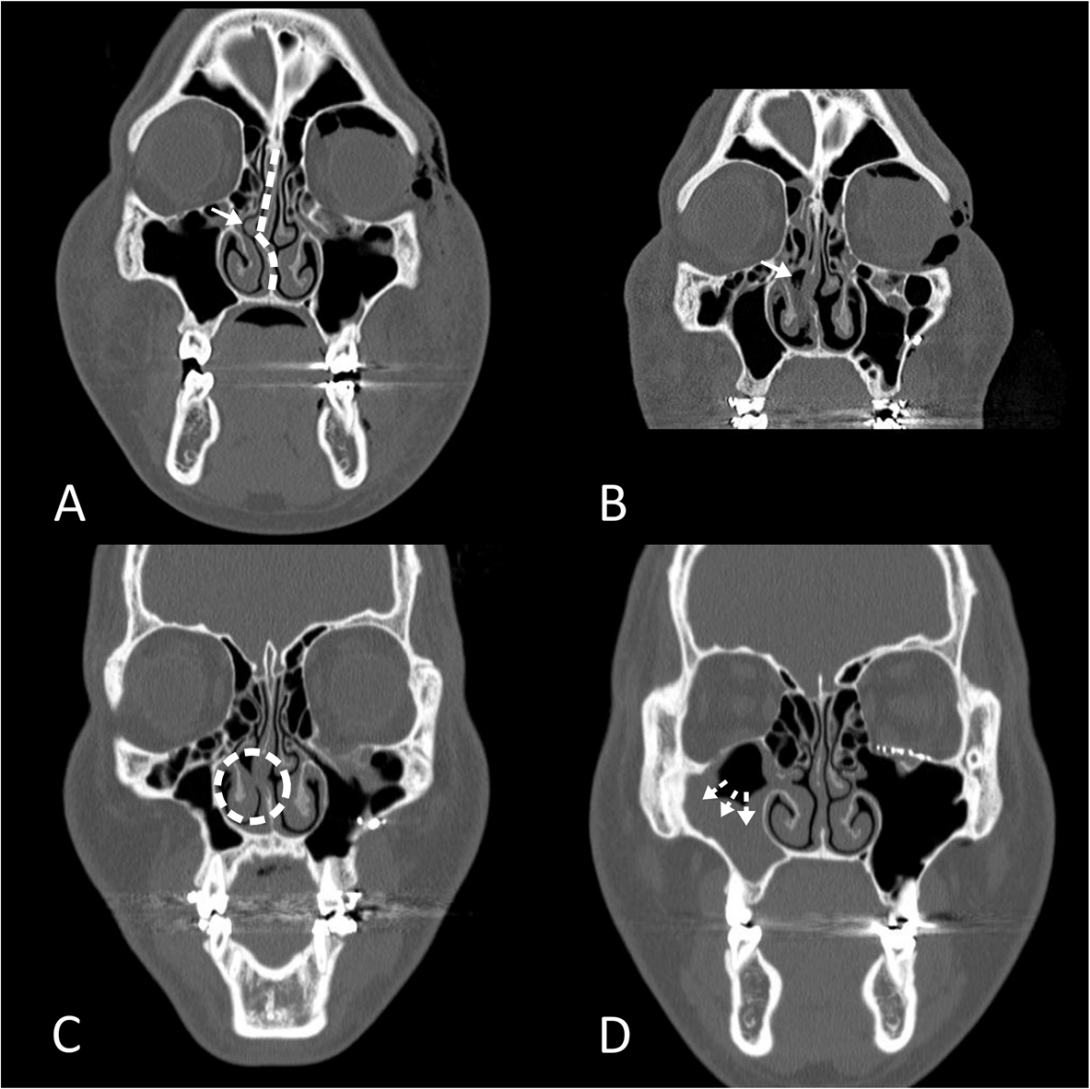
Facial computed tomography (CT) scans of the nose. a Preoperative CT scan; right deviated nasal septum is noted (dotted line). b One-day postoperative CT scan; absence of right middle turbinate is noted (White arrow on panel A and B). c Three-month postoperative CT scan; formation of synechiae is noted in right nasal cavity (dotted circle). d Two-year postoperative CT scan; right maxillary sinusitis is noted (dotted arrow).
